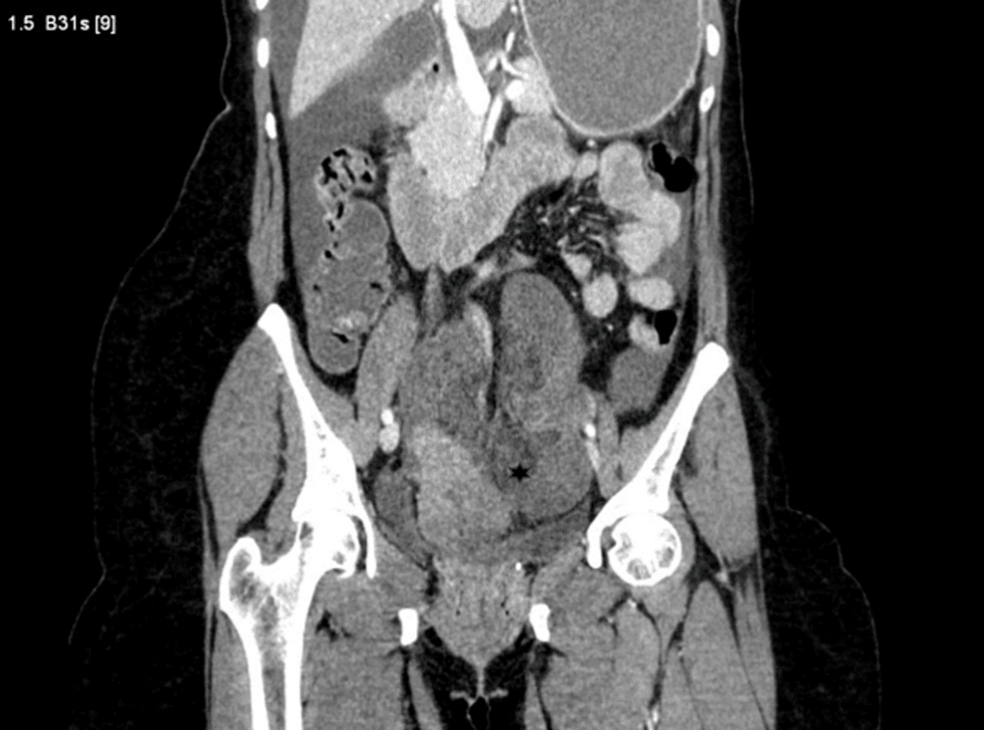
Computed tomography showing non-enhancing ileal loops. Coronal plane computed tomography demonstrating gangrenous changes of ileal loops within the pelvis (*) and mild dilatation of proximal small bowel loops. Additionally, a notable amount of moderate fluid was observed within the peritoneal cavity.
